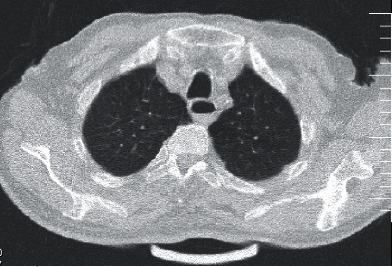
Male patient's chest CT.
